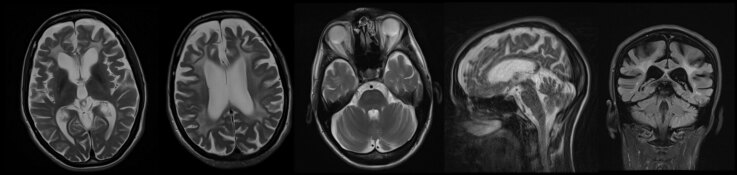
Bilateral symmetrical T2-weighted/FLAIR hyperintensities, together with global brain atrophy in patient carrying pathogenic variant in *ARSA* gene (Case 1).
